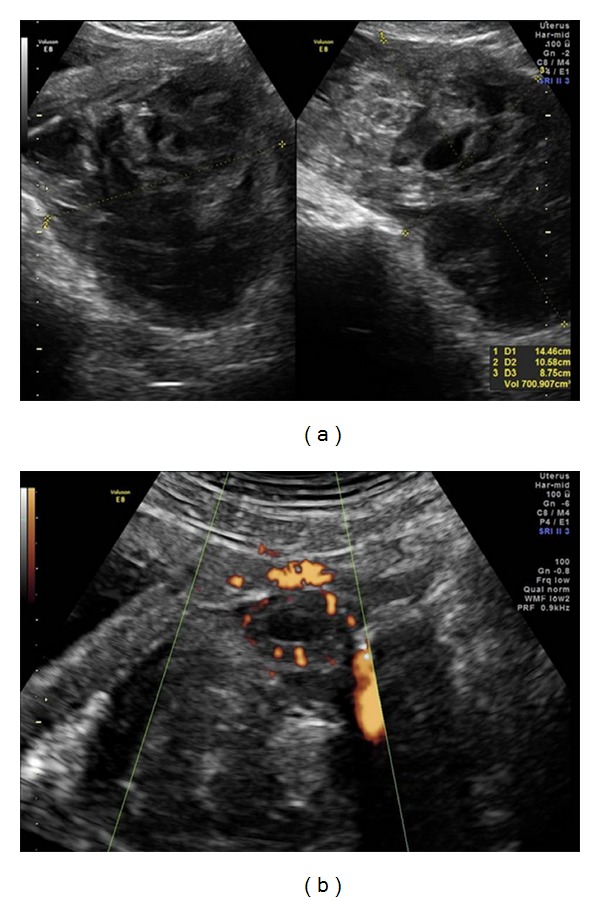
(a) B-mode endovaginal 2DUS showing a large irregular heterogeneous mass in the left pelvic abdominal region; (b) endovaginal 2DUS in power Doppler mode showing “ring” vascularization, indicative of a gestational sac. 2DUS: two-dimensional ultrasound.
